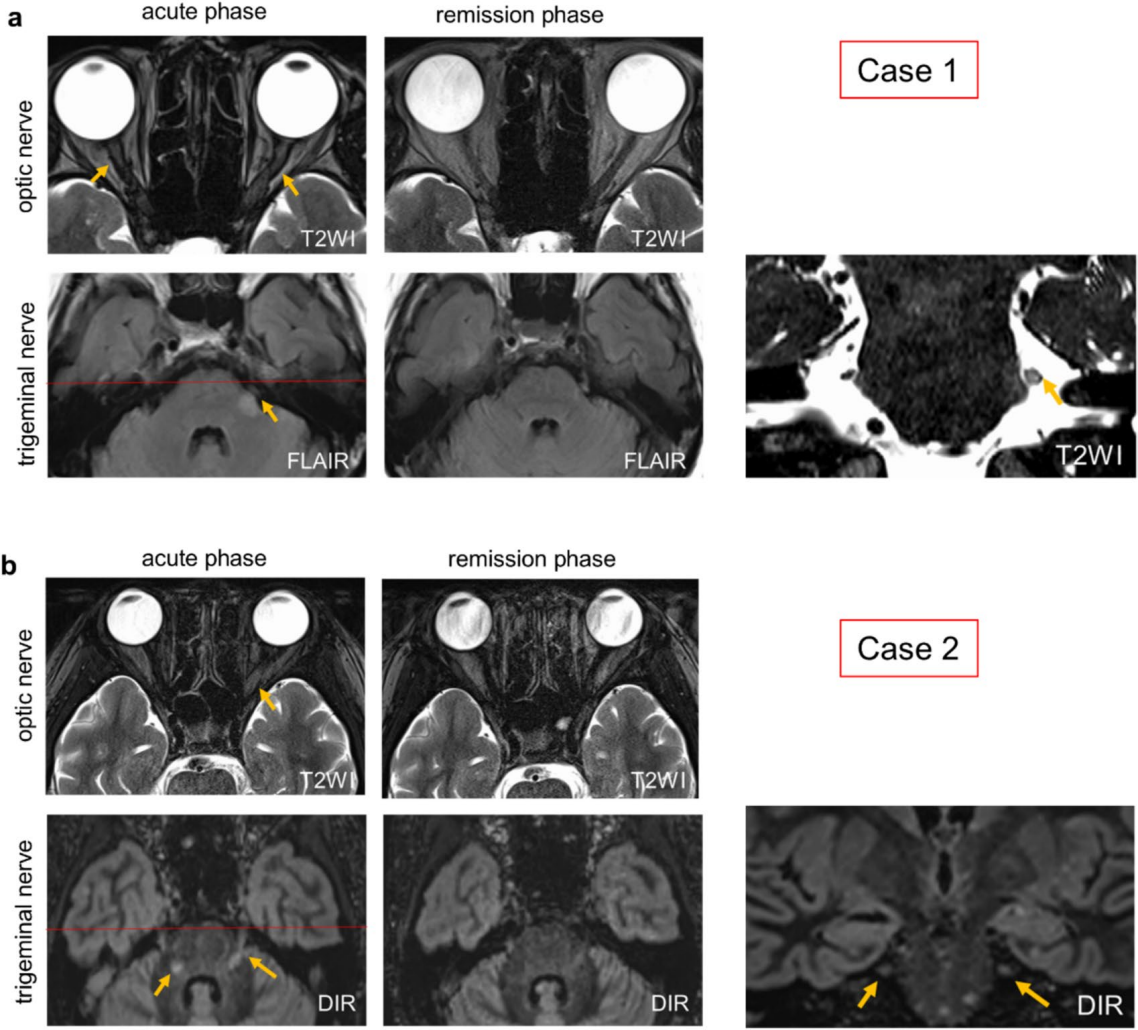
Two patients demonstrating both optic neuritis and trigeminal nerve lesions. a Axial T2 weighted images (T2W1) of the optic nerves showed bilateral hyperintensities (yellow arrows) at acute phase, which completely resolved at the remission phase in case 1 (a patient with inflammatory demyelinating disease of undefined type). Fluid attenuated inversion recovery (FLAIR) sequences showed a high signal intensity at the REZ of the left trigeminal nerve of this patient (yellow arrow) at acute phase, which partially resolved at remission phase. b Axial T2WI images showed a hyperintensity lesion of the left optic nerve at acute phase of case 2 (a MOGAD patient), which was nearly completely resolved at remission phase. The double inversion recovery (DIR) sequences showed bilateral lesions at the pons and the REZ of the trigeminal nerves that extended from the pons distally up to 4 mm. The trigeminal nerve lesions were partially resolved at remission phase. Coronal views (right panels) show the red scanned line corresponding to the axial view at the acute phase
